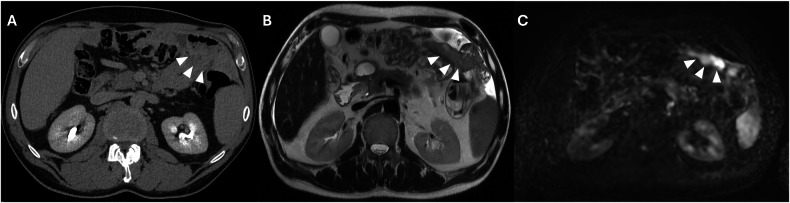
Gastric cancer with peritoneal carcinomatosis in an 81-year-old man. CT delayed phase (A), MRI T2w and DWI (b = 800 mm^2^/s) (B, C) axial plane showed a large implant known as a “Plaque-like” pattern (arrowheads).Omental cake: large plaques surrounded by reactive fibrotic tissue that results in the consolidation of omental fat, which appears stratified and causes posterior displacement of the bowel relative to the anterior abdominal wall (Fig. 7) [29].
